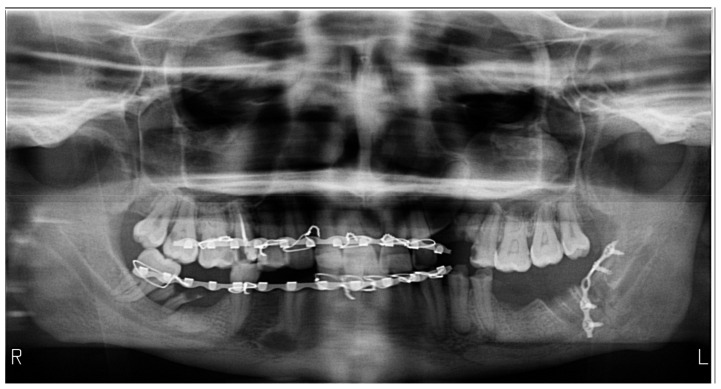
Orthopantomography with a sad appearance, a blurred frontal region, produced by the head extension position, in a patient treated surgically for a fracture of the left angle of the mandible. The asymmetry between the right and left sides with a larger hemi-mandible on the right side is produced by a lateral deviated position of the head towards the left side. Additionally, superior apical portions in the frontal region are obscured by an air band in the palatoglossal space. The bite-block was not used due to bimaxillary immobilization.
